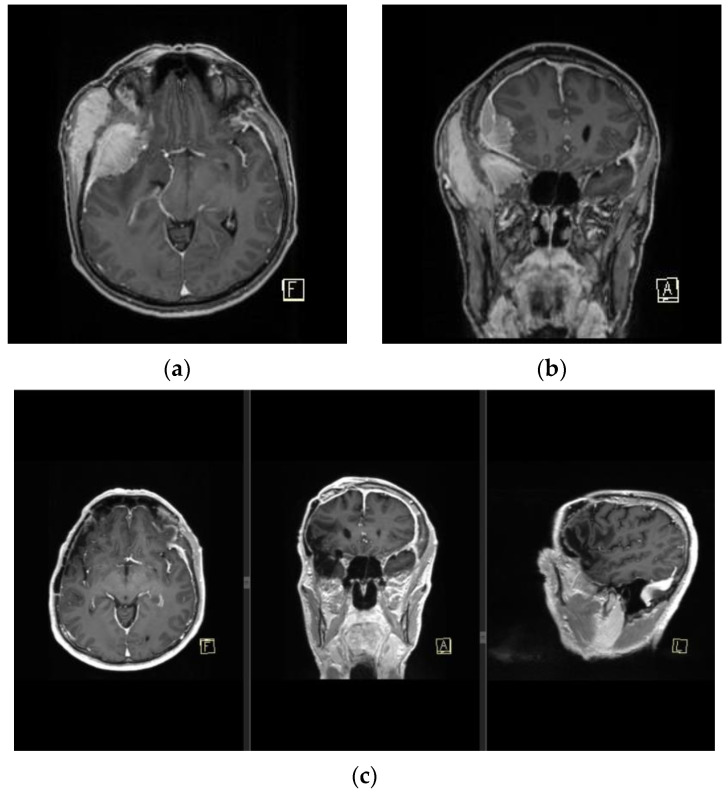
MRI finding of pan-meningiomatosis. (a,b) show preoperative MRI findings of an invasive anaplastic menigioma in a RIM patient due to tinea capitis radiation treatment in childhood, and prior to a wide Simpson gr I tumor resection with duroplasty and cranioplasty. (c) shows a postoperative MRI finding of the patient with a complete dural signal enhancement.
